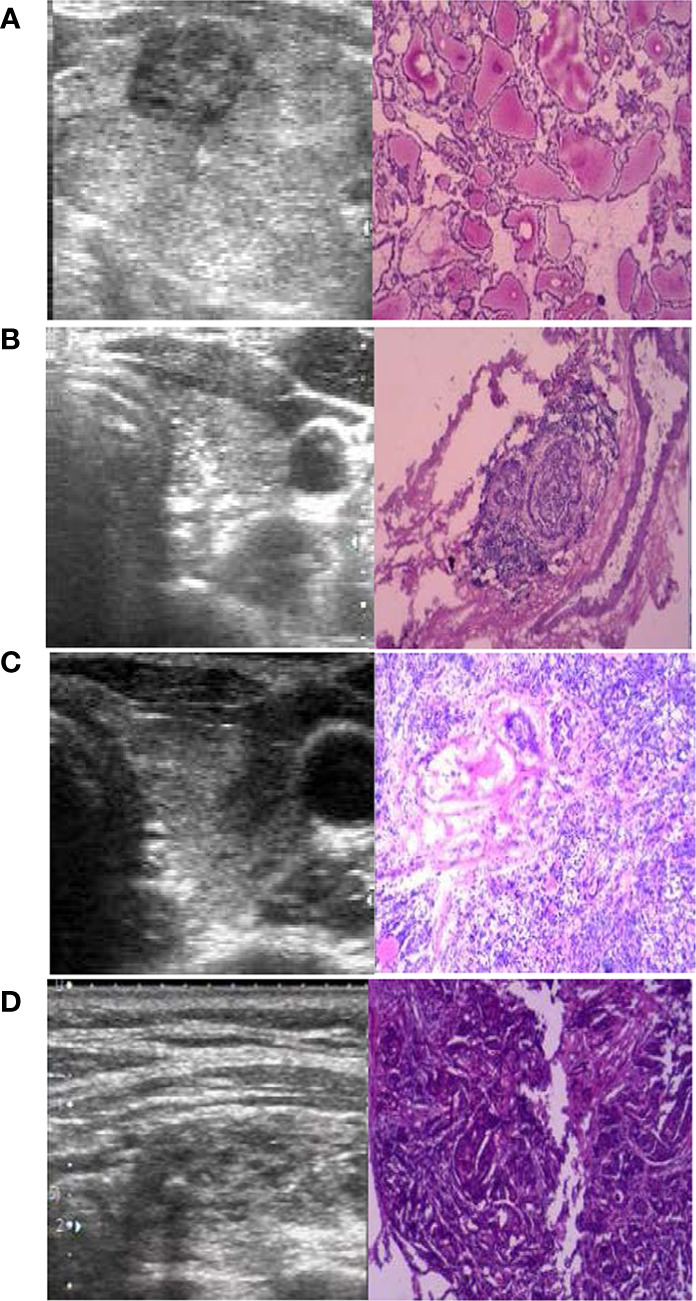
The preoperative ultrasound (US) and postoperative pathology (×100). (A) The ablation region had a new PTC four years after radiofrequency ablation (RFA). (B) The lymph nodes were metastasis (positive/all was 7/9) 3 months after RFA. (C) The PTC was not completely ablated 3 months after RFA. (D) The PTC was completely ablated 1 months after RFA.
